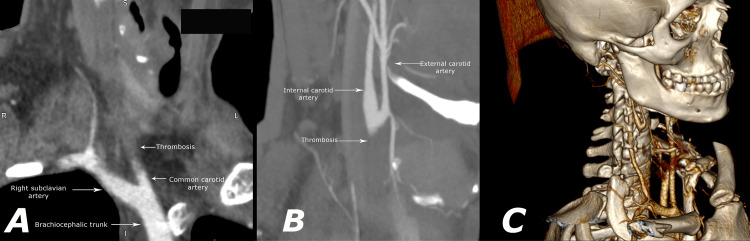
А and B) Preoperative CT angiogram (CTA) showing complete occlusion of the right common carotid artery with decreased collateral filling of the right internal carotid and middle cerebral artery; C) 3D reconstruction based on the CTA providing volumetric representation of the data
